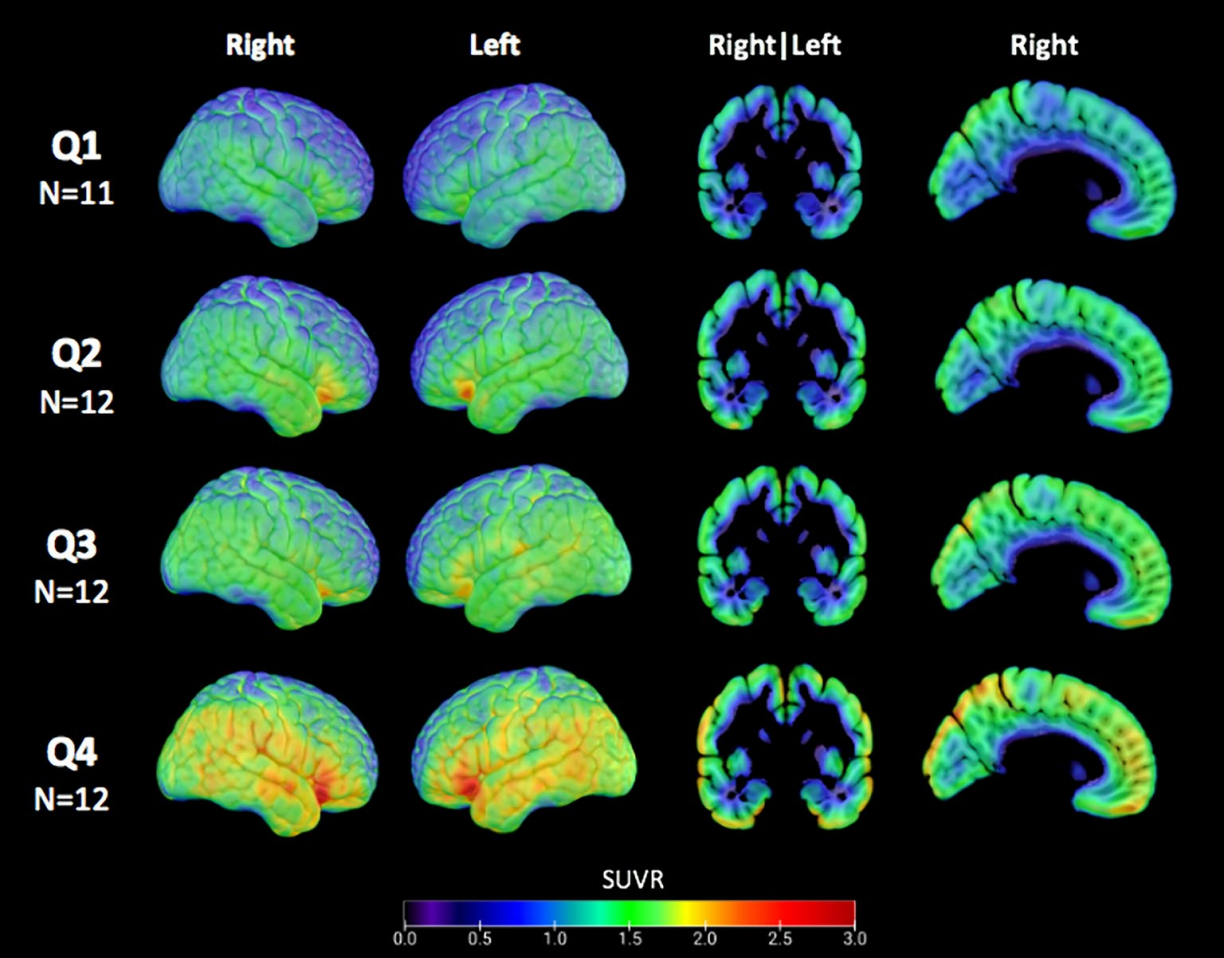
Mean tau-PET SUVR values across quartiles
